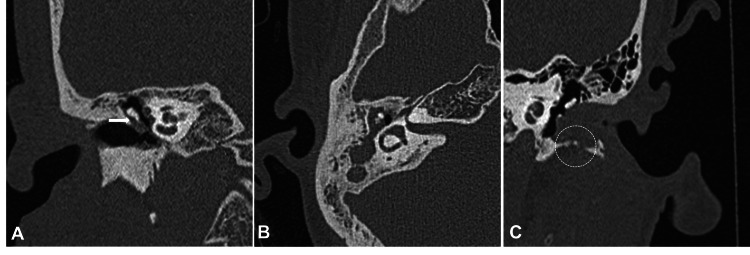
HRCT images demonstrating locations of cholesteatoma. Coronal reformatted HRCT image of the right temporal bone (A) shows non-dependent soft tissue in Prussak’s space (white arrow) proved as cholesteatoma. Axial HRCT image of right temporal bone showing attico-antral cholesteatoma (B). Coronal reformatted HRCT image demonstrating soft tissue density lesion and bony erosions in the left EAC (C), a rare cholesteatoma location. EAC: external auditory canal, HRCT: high-resolution computed tomography
